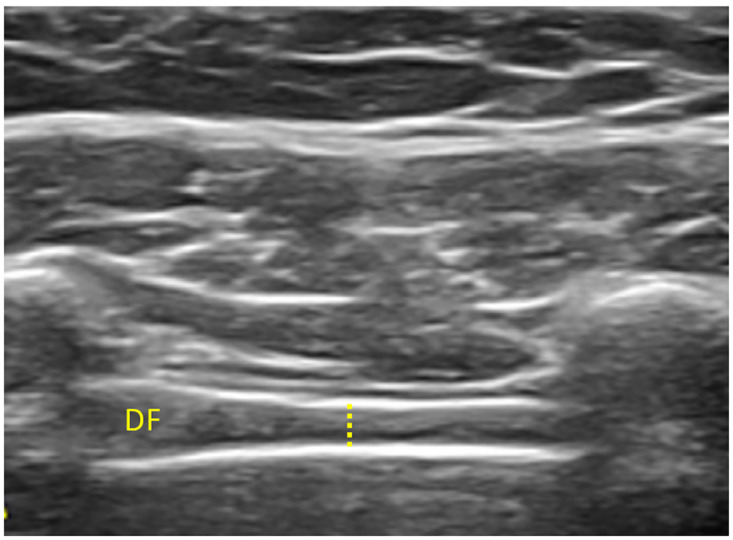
Ultrasonography of the diaphragm muscle.
